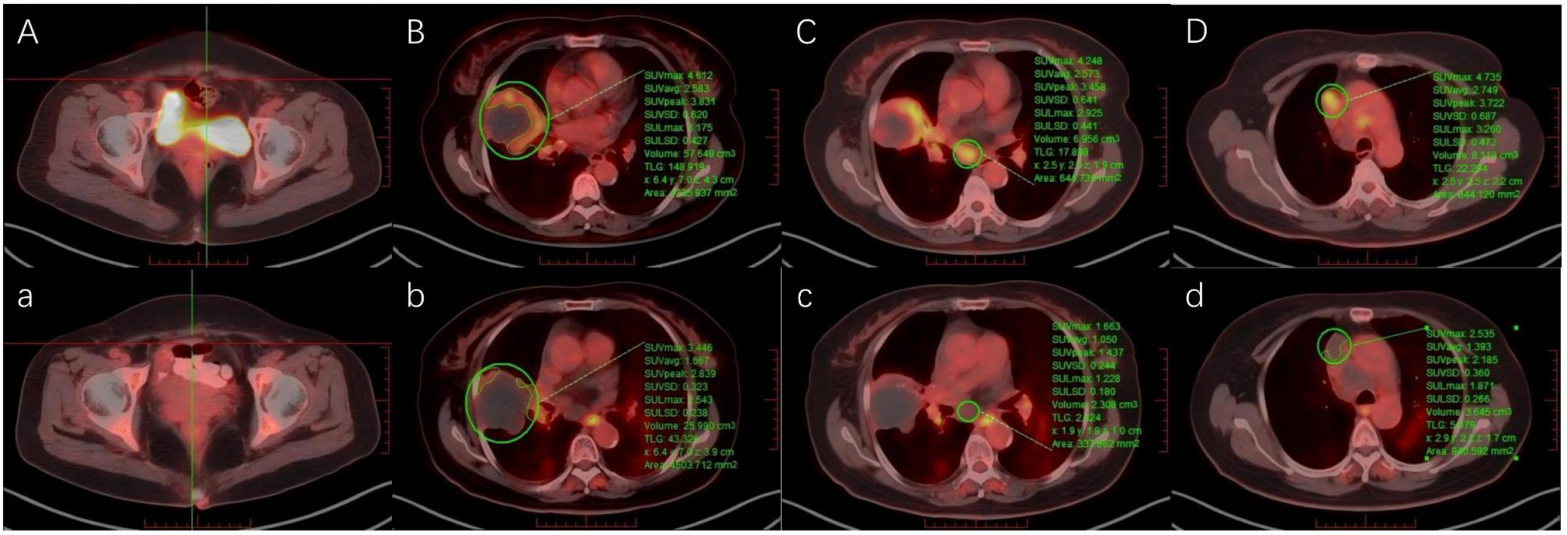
Pre-treatment and post-treatment ^18^F-FDG PET-CT images of (A, a) the surgical site, (B, b) the right lung lesion, (C, c) mediastinal lymph nodes in station 7, and (D, d) mediastinal lymph nodes in station 3.
